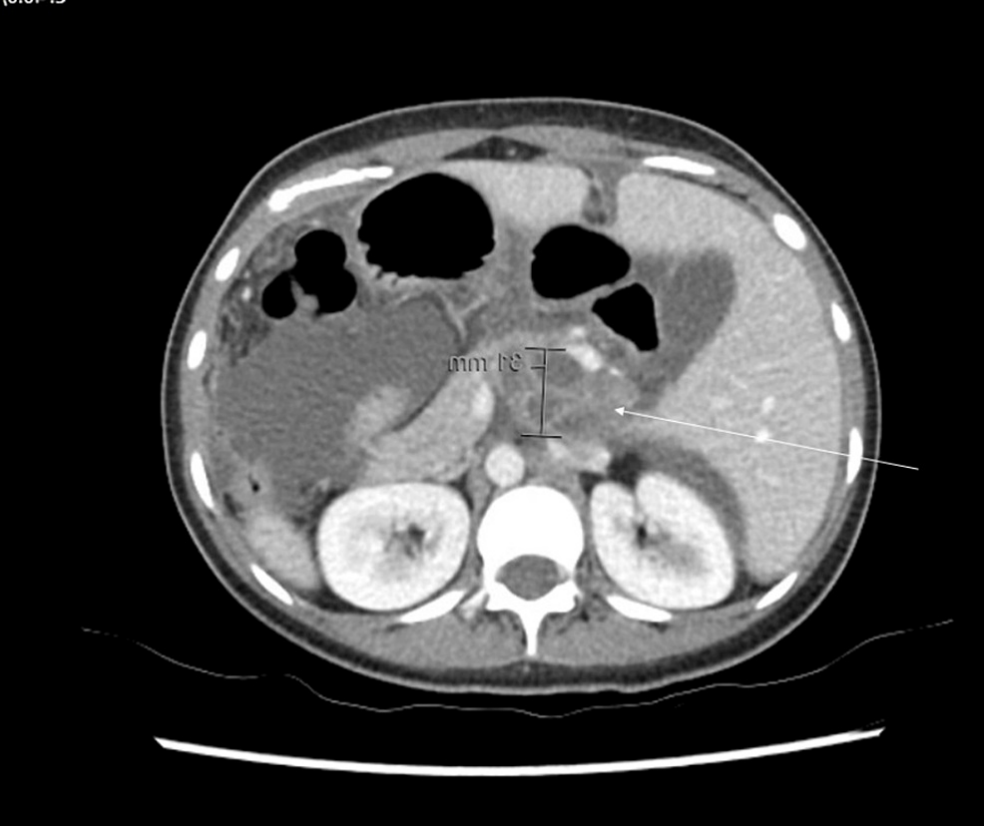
Axial computed tomography image of a multiloculated cystic lesion (3x4.7x5cm) (white arrow) in the upper abdomen between the portal vein and inferior vena cava and between the pancreatic head and caudate lobe of the liver
